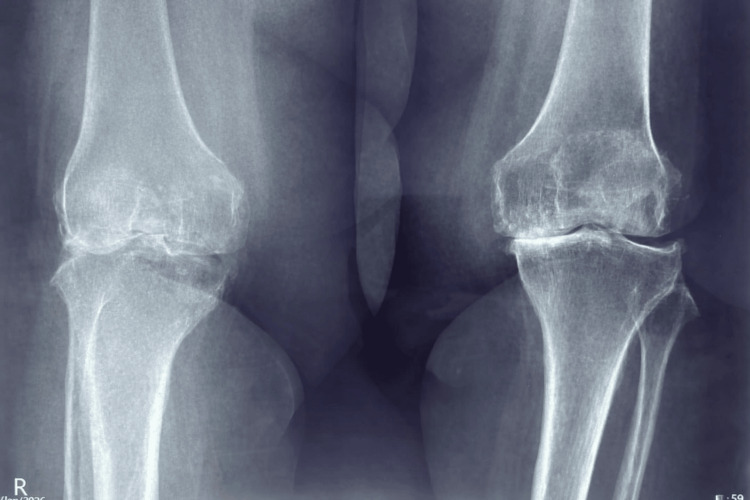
Preoperative standing anteroposterior radiograph of both knees X-ray demonstrating advanced degenerative osteoarthritis characterized by joint space narrowing, osteophyte formation, and subchondral sclerosis.
